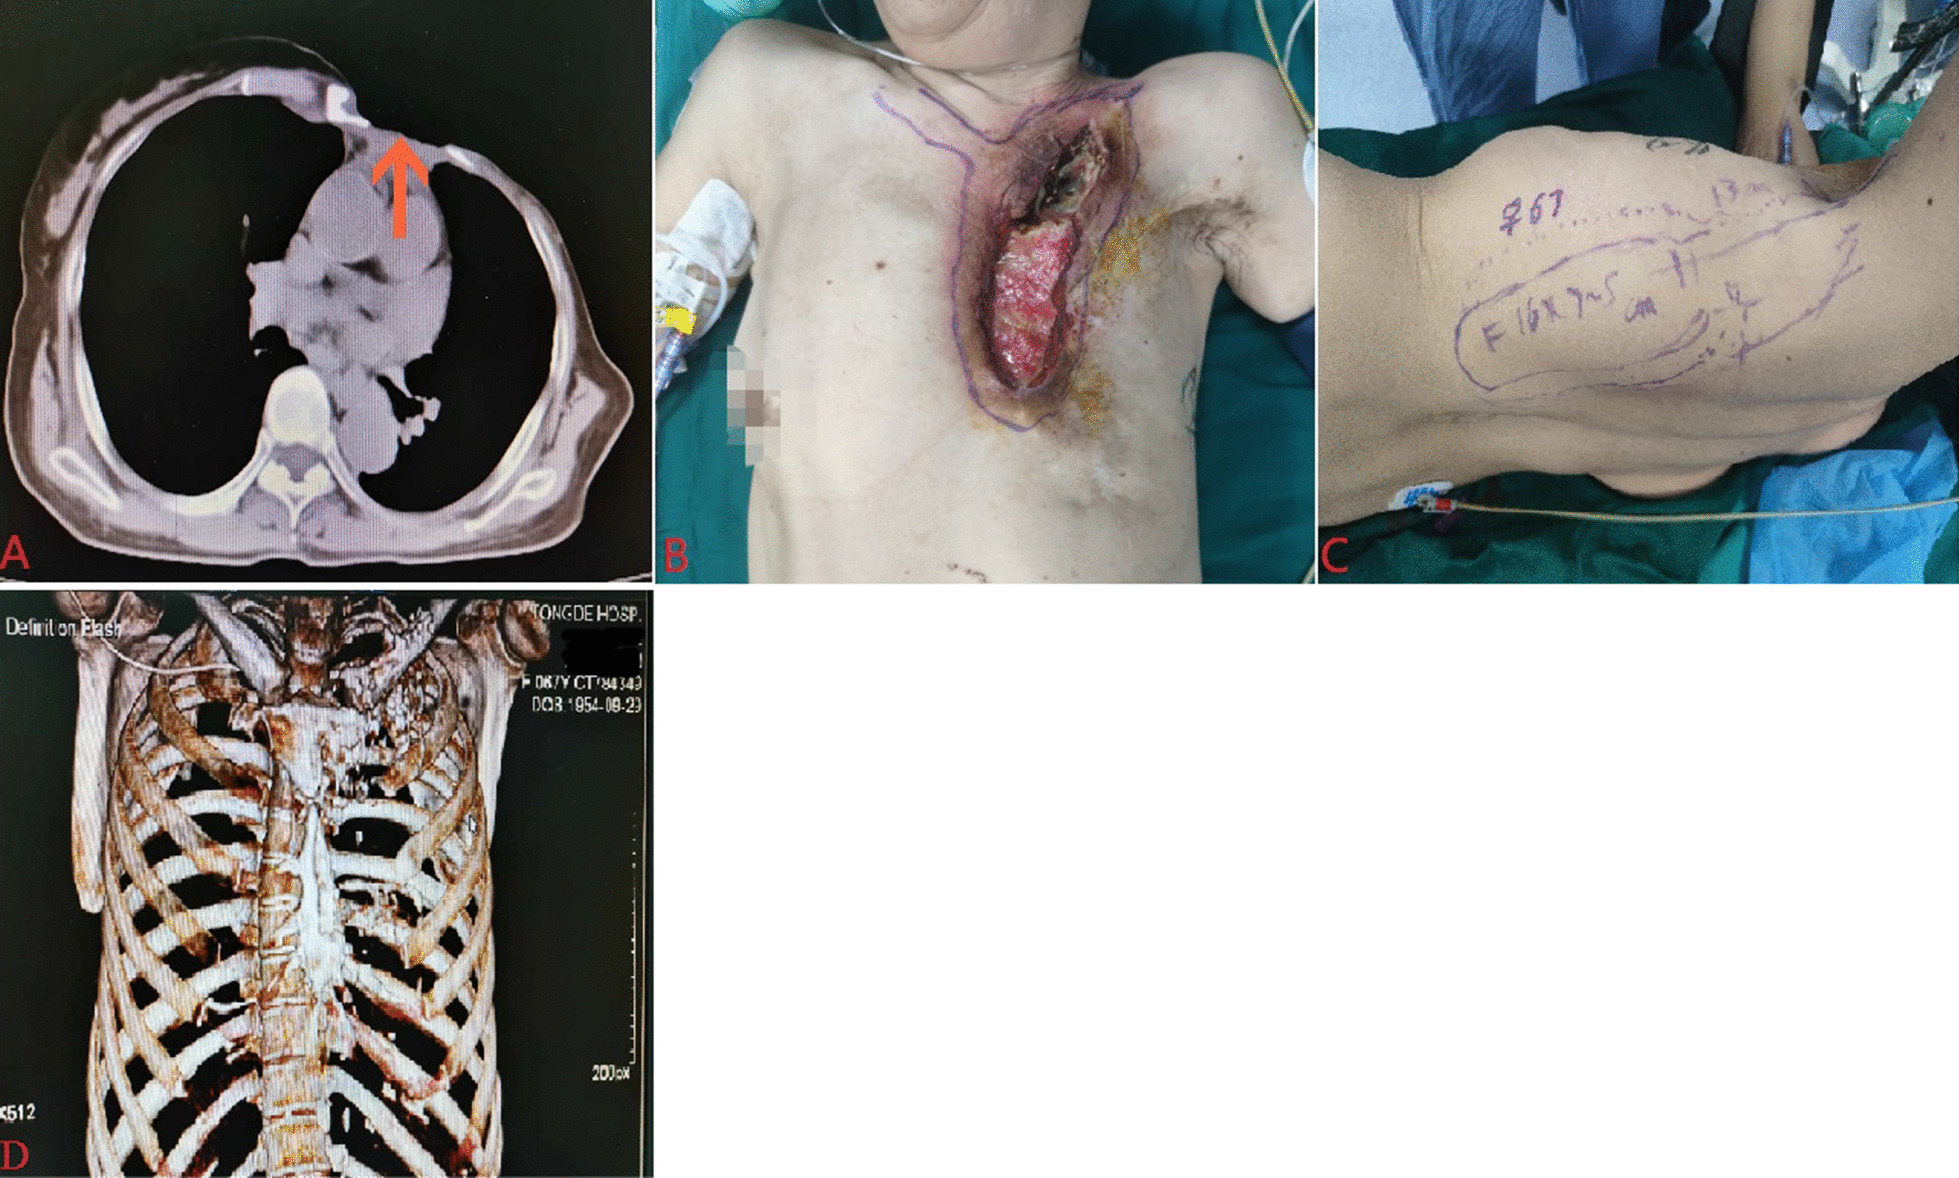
General information of patients before surgery. A Chest CT showed that the patient had a huge defect in the left anterior chest wall and part of the bone had been destroyed (Orange arrow). B After first-stage operation debridement of the left anterior chest wall sinus, the changes involved the sternum, rib and clavicle, and proliferative fresh granulation tissue was seen in some wound areas. C The range of pedicled latissimus dorsi myocutaneous flap was designed before operation. D Preoperative 3-D imaging showed high-density shadow and destruction of left clavicle, part of ribs and part of sternum
